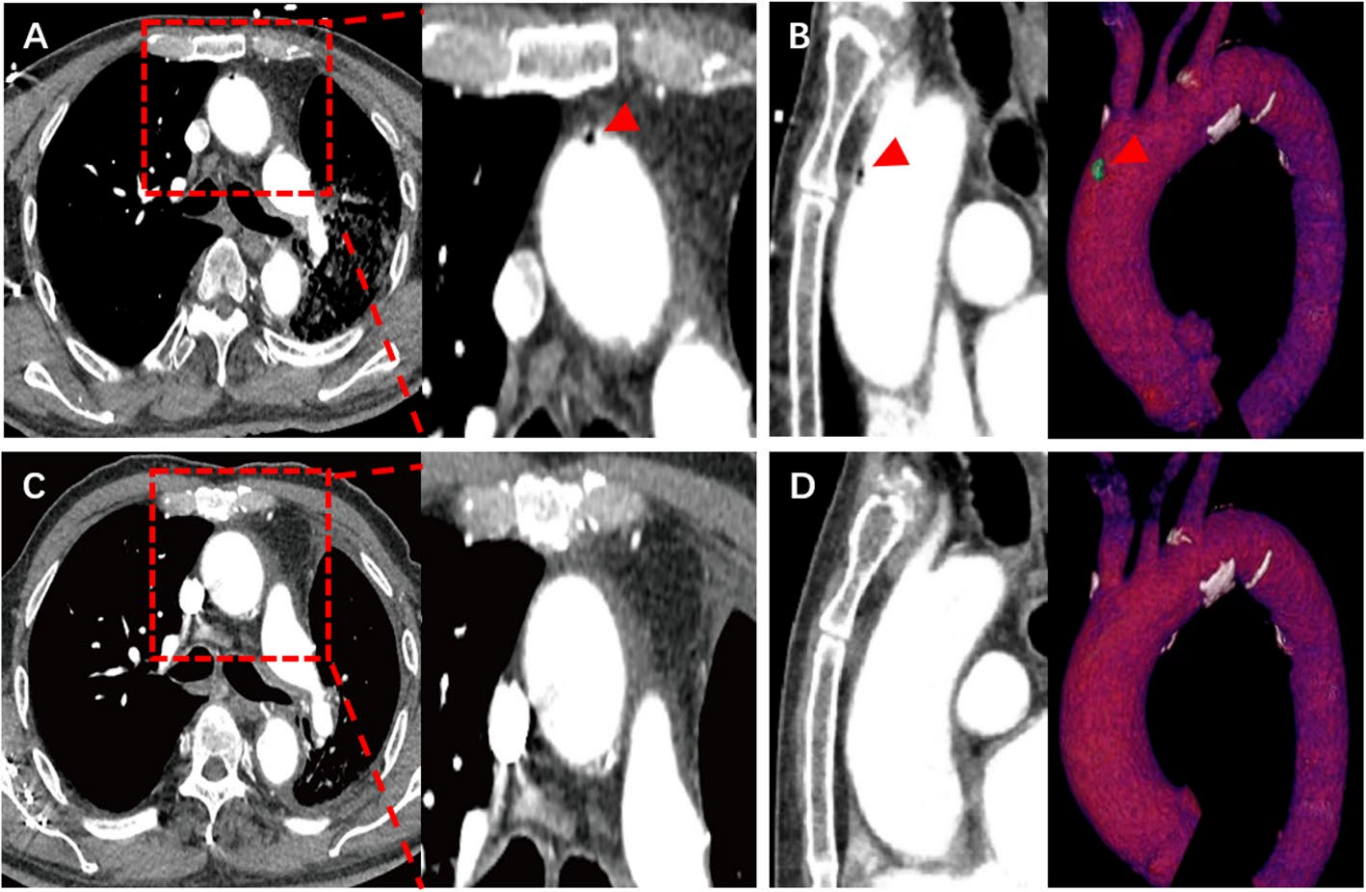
Bubbles in aorta on postoperative chest CTA. A Bubbles in the aorta on raw chest CTA (red arrow). B Bubbles in the aorta on three-dimensionally reconstructed chest CTA (red arrow). CTA = computed tomography angiography
